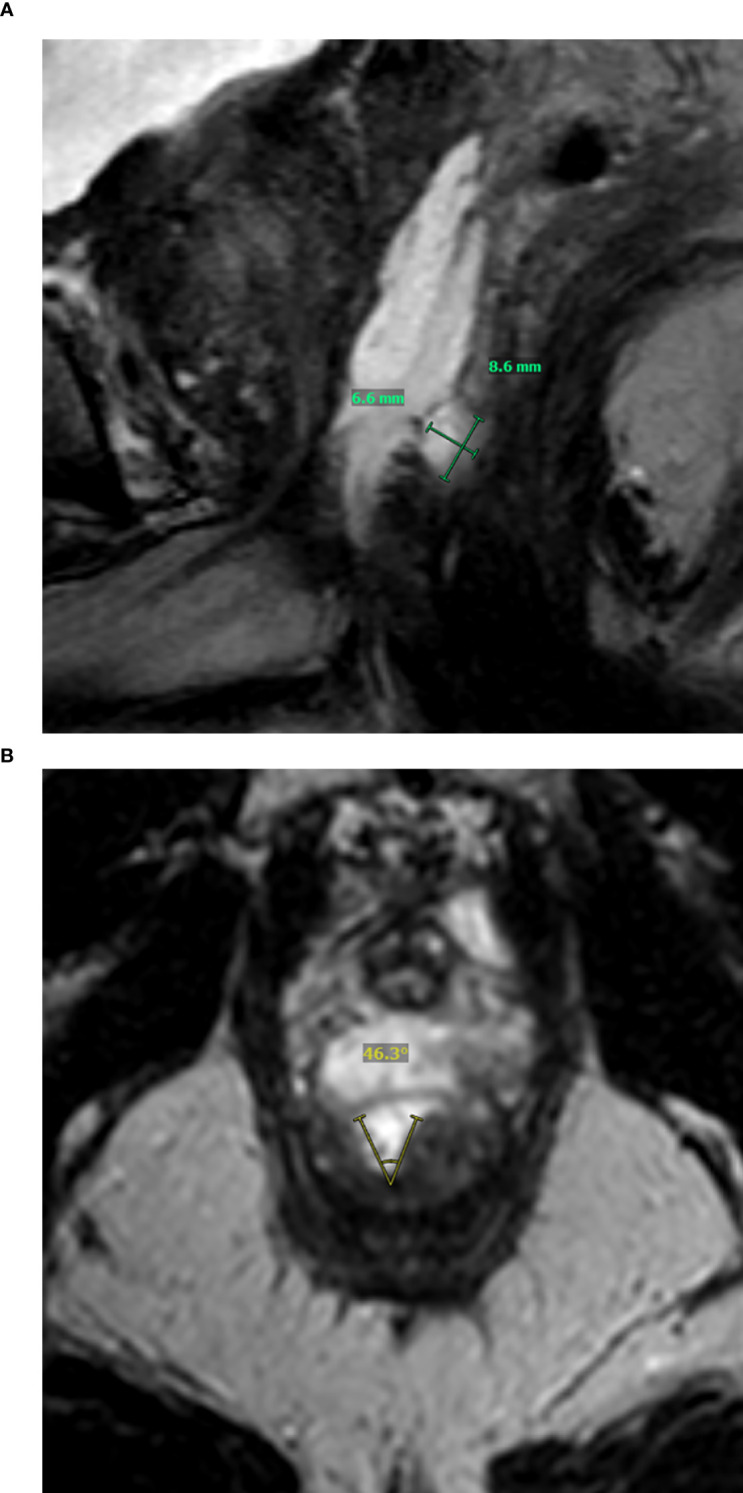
Representative example showing assessment of location and extent of rectal wall infiltration (RWI) on MRI. (A) Sagittal T2-weighted imaging shows deep RWI (score 3) at the apex with radial depth of 6.6 mm and longitudinal length of 8.6 mm. (B) Axial T2-weighted imaging shows that RWI involves 46.3°C (12.9%) of the rectal circumference. RWI, rectal wall infiltration.
